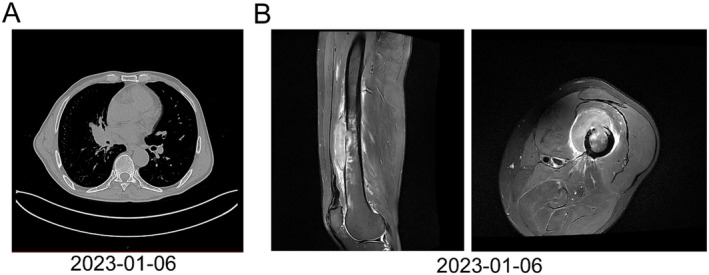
Enhanced computed tomography (CT) scans. (A) Chest scans indicated the presence of a right hilum nodule in the lung. (B) Leg scans showed tumor infiltration in the femur.
